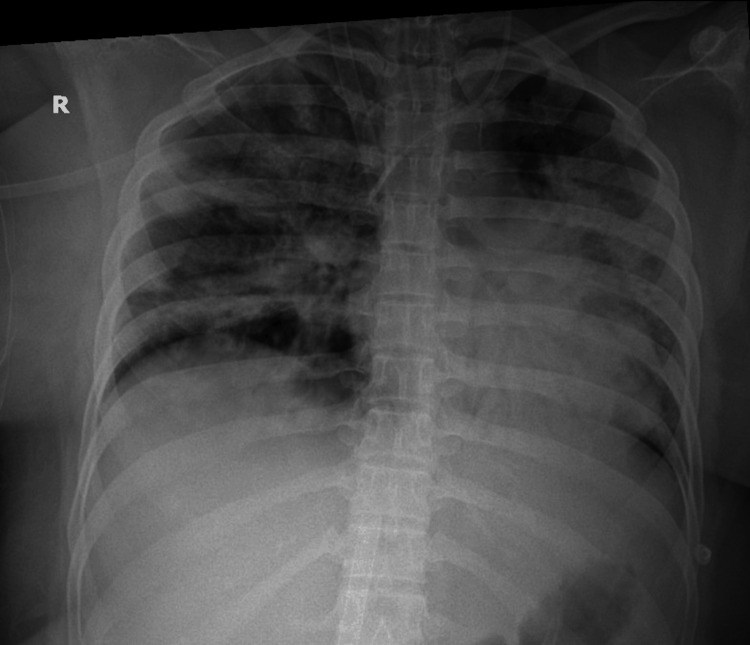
Worsening chest X-ray on day 9.
